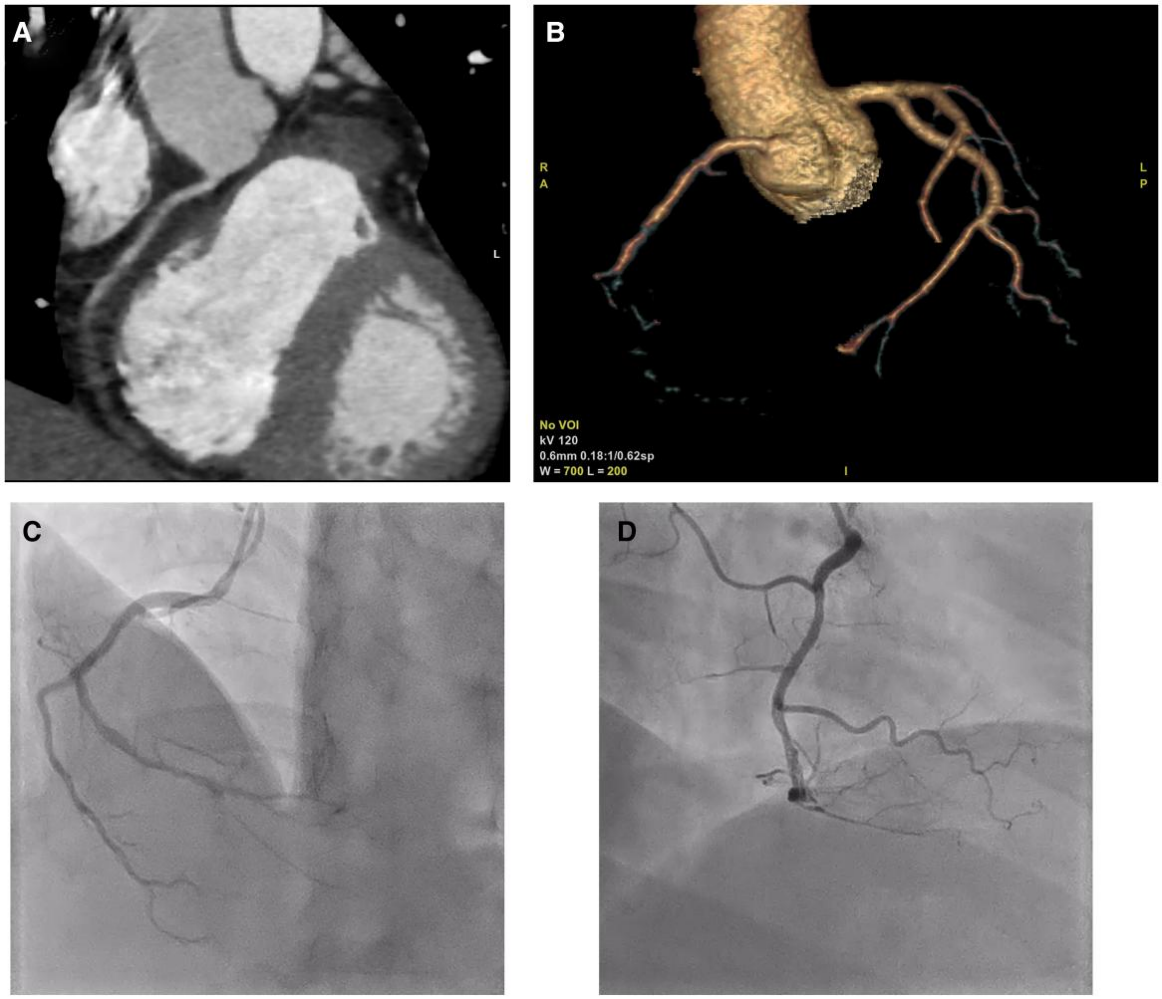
Follow-up coronary angiography of right coronary arteries after 1 year and 4 months (*A*, *B*) contrast-enhanced computed tomography of right coronary arteries on Day 24. Percutaneous coronary intervention was performed on Day 9, but thrombotic occlusion recurred from the proximal right coronary arteries on Day 24. (*C*, *D*) Coronary angiography of the right coronary arteries. An improved coronary blood flow was confirmed via coronary angiography when compared with the contrast-enhanced computed tomography of the coronary arteries performed on Day 24.
